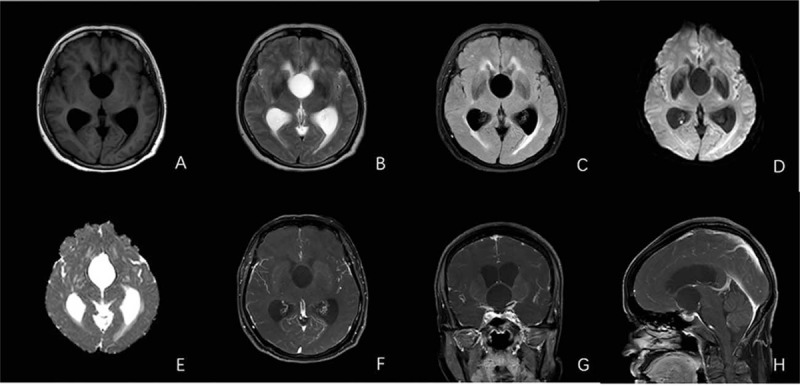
MRI of the patient's brain and pathological results. (A–E) MRI of the brain shows a cystic long T1/T2 signal intensity in the suprasellar area. (F–H) Enhanced MRI of the brain shows clear margins of the lesion and no cyst wall enhancement and internal lesion. MRI = magnetic resonance imaging
